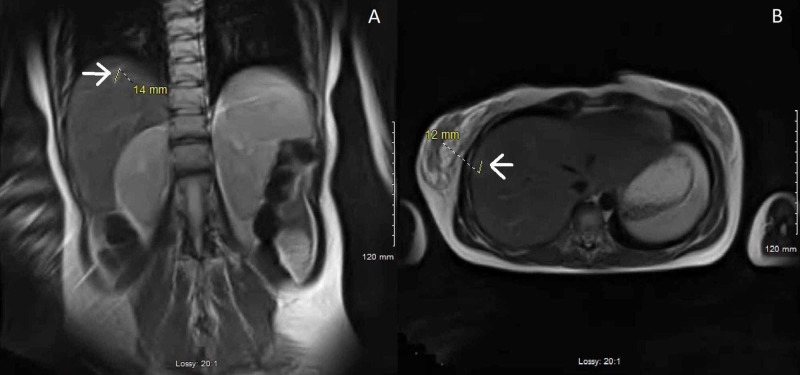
Magnetic resonance imaging (A, B) Two non-specific hepatic lesions.
